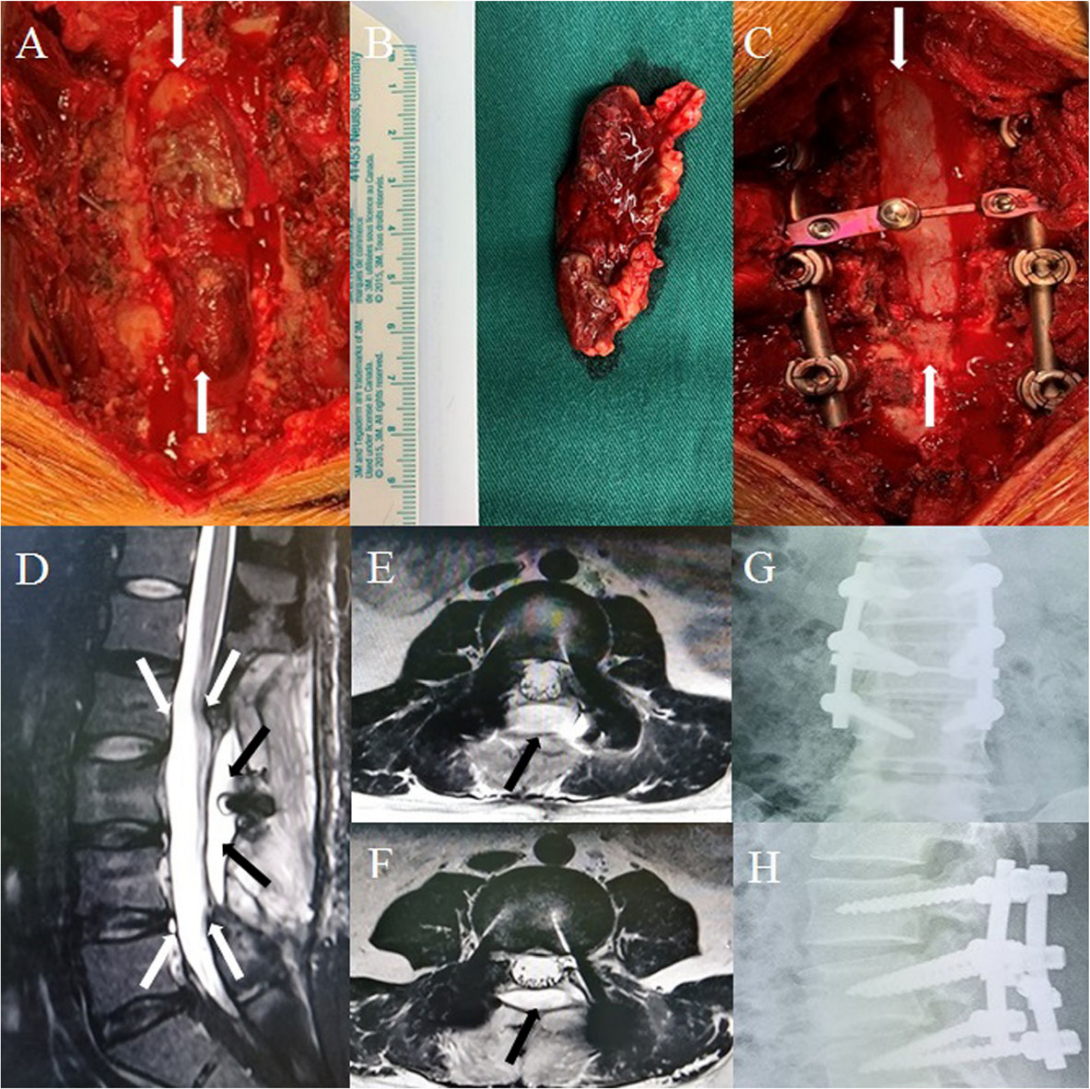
a The epidural soft mass in the vertebral canal during surgery. The white arrows show the scope of the mass. b The approximately 6.0 × 2.5 × 1.2 cm monolithic irregular fusiform soft mass was completely resected. (c) The restored dura sac became engorged and pulsatile after the mass resection. The white arrows show the scope of the dura sac. The postoperative 3rd month magnetic resonance images (MRI) and X-ray images. d T2-weighted MRI sagittal and (e) (f) transverse views show the dura sac was engorged without any compression. The white arrows show the scope of the engorged dura sac. The black arrows show the postoperative soft tissue changes. No obvious mass relapse was observed. The anteroposterior X-ray image (g) and lateral image (h) show the screw-rod fixation was normal
